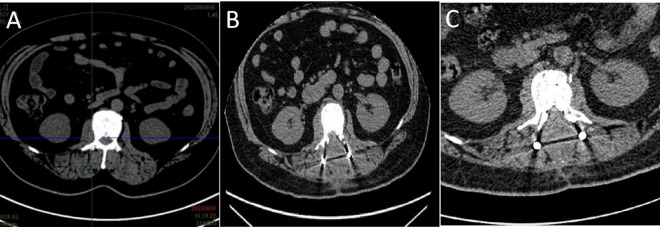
(A) PET/CT scan at the L2 level before surgery. (B) CT scan at the L2 level after surgery. (C) CT scan at the L2 level after one cycle of retinoic acid combined with arsenic trioxide induction therapy.
